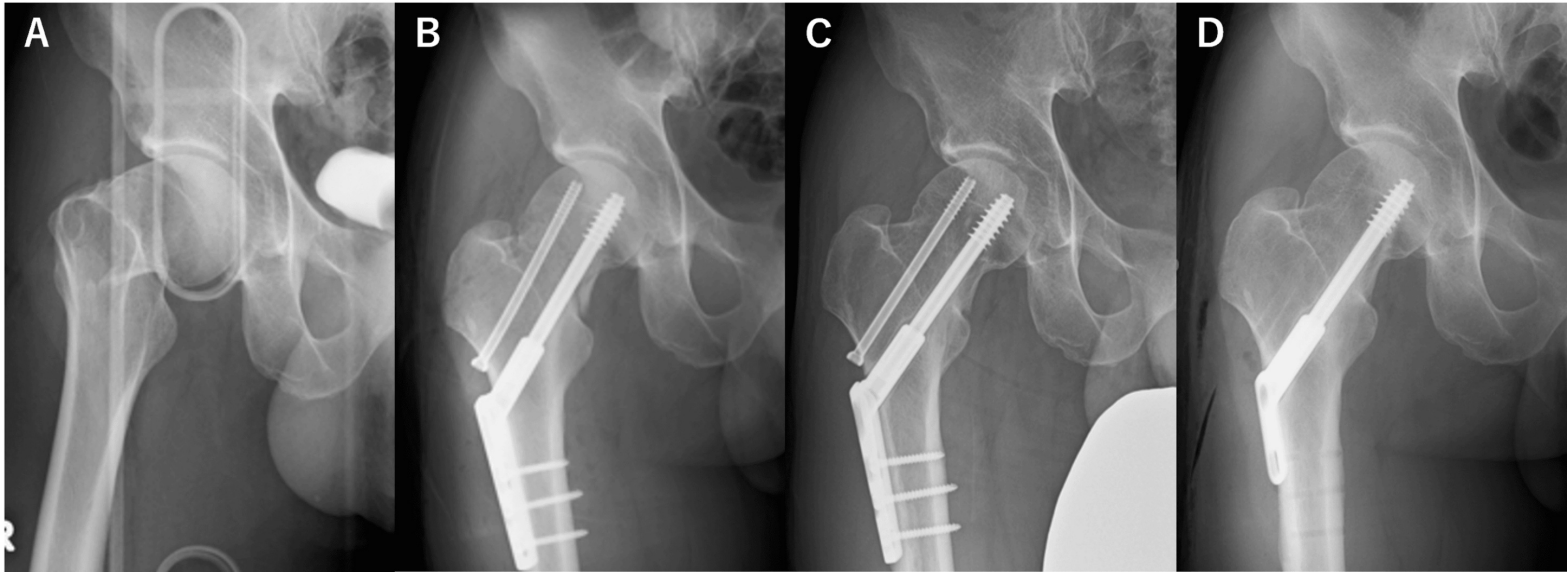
X-rays images of a 31-year-old male patient with femoral neck fracture (Garden stage IV). (A) Initial radiograph. (B) Radiograph at internal fixation with a keyless-type CHS system. (C) Radiograph at 15 months after internal fixation. (D) Radiograph after implant removal. The lag screw could not be removed due to strong fixation in the femoral head. CHS: compression hip screw
